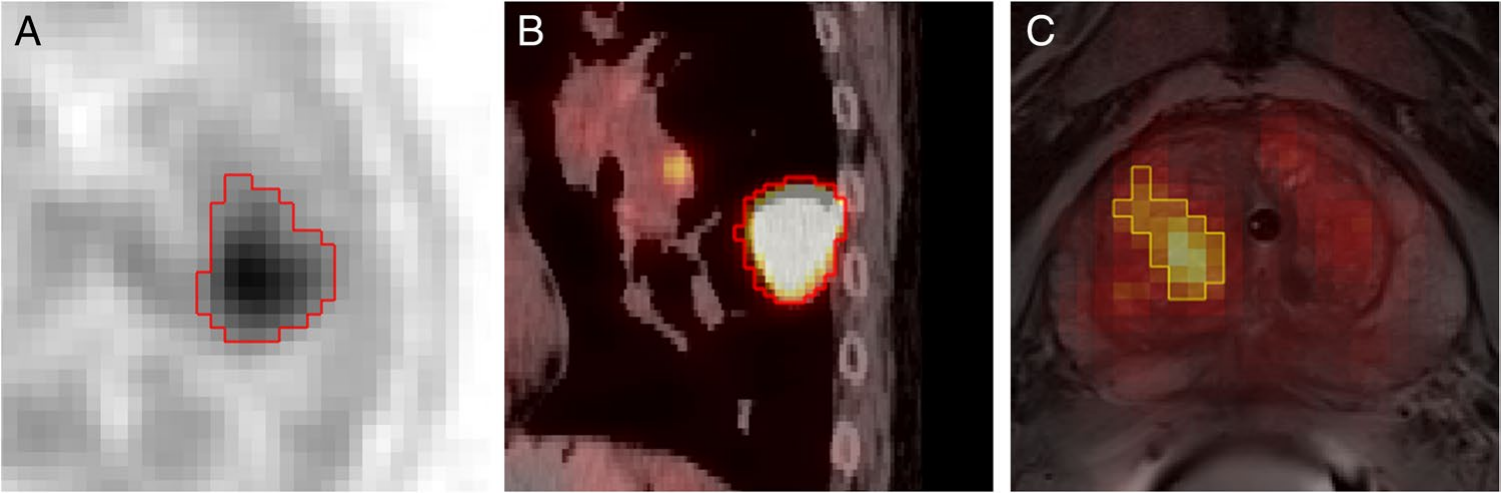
Manual delineation (Ref-B) example views of the cohorts involved in this study. A Glioma tumor (axial view, inverted gray palette with range 0–7 SUV); B Lung tumor (sagittal view, PET hot metal palette with range 0–5 SUV, CT gray palette with range -100.200 HU); C Prostate tumor (oblique-axial view, PET hot iron palette with range 0–9 SUV, T2w MRI grey palette). PET—Positron Emission Tomography; SUV—Standard Uptake Value; T2w—T2-weighted magnetic resonance imaging (MRI)
